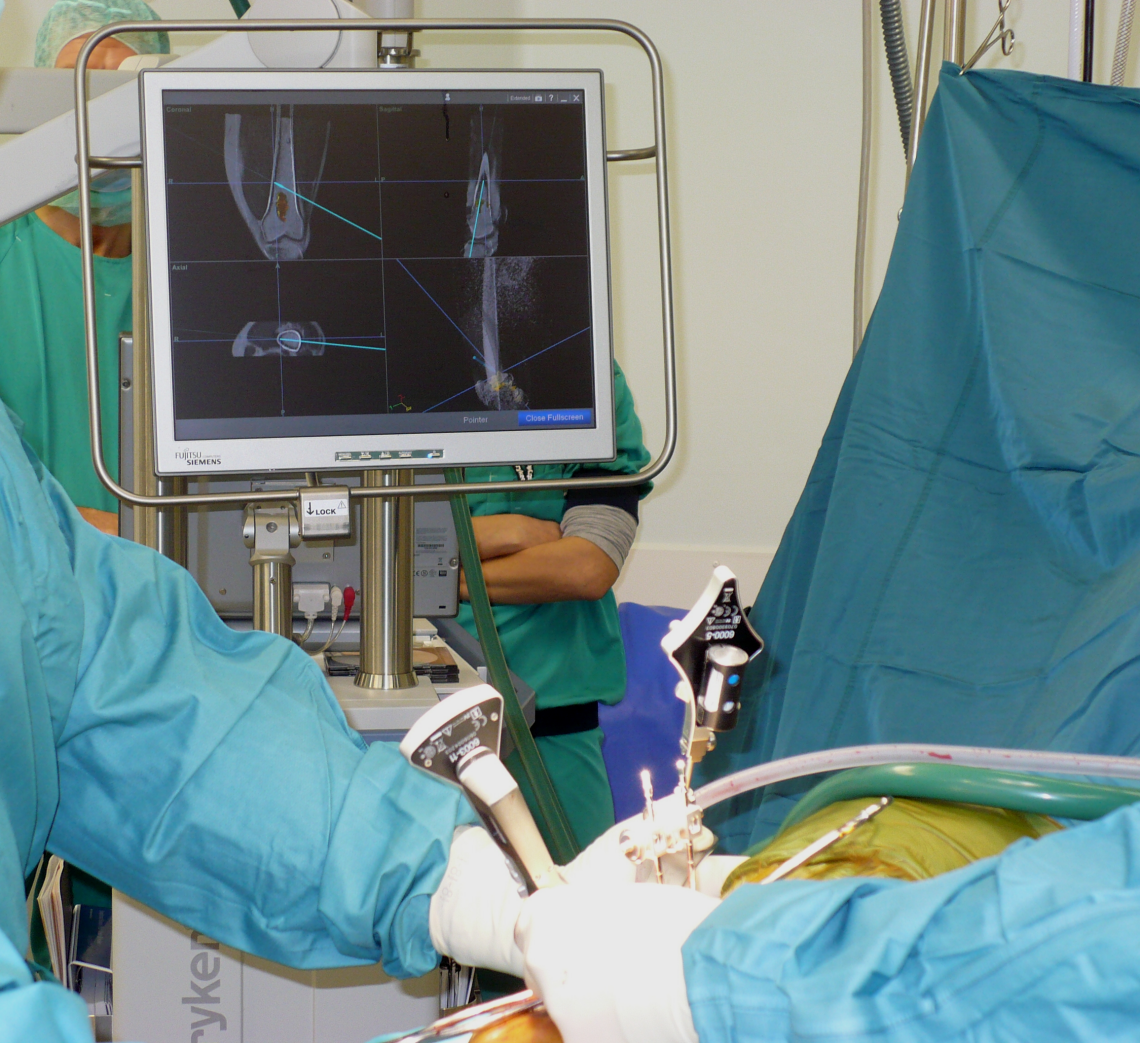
CAS procedure. Photograph during a typical CAS supported curettage procedure. Imagebased visualization mode is set to standard view. The system shows the relevant MRI slices, fused to the CT dataset, based on position of the tool. The ACT/CS1lesion is colored yellow by manual segmentation. The curette is visible as the blue line and the blue dotted line shows its vector in each direction. The lower right screen shows a volume render of the dataset, with the curette as the blue tool.
